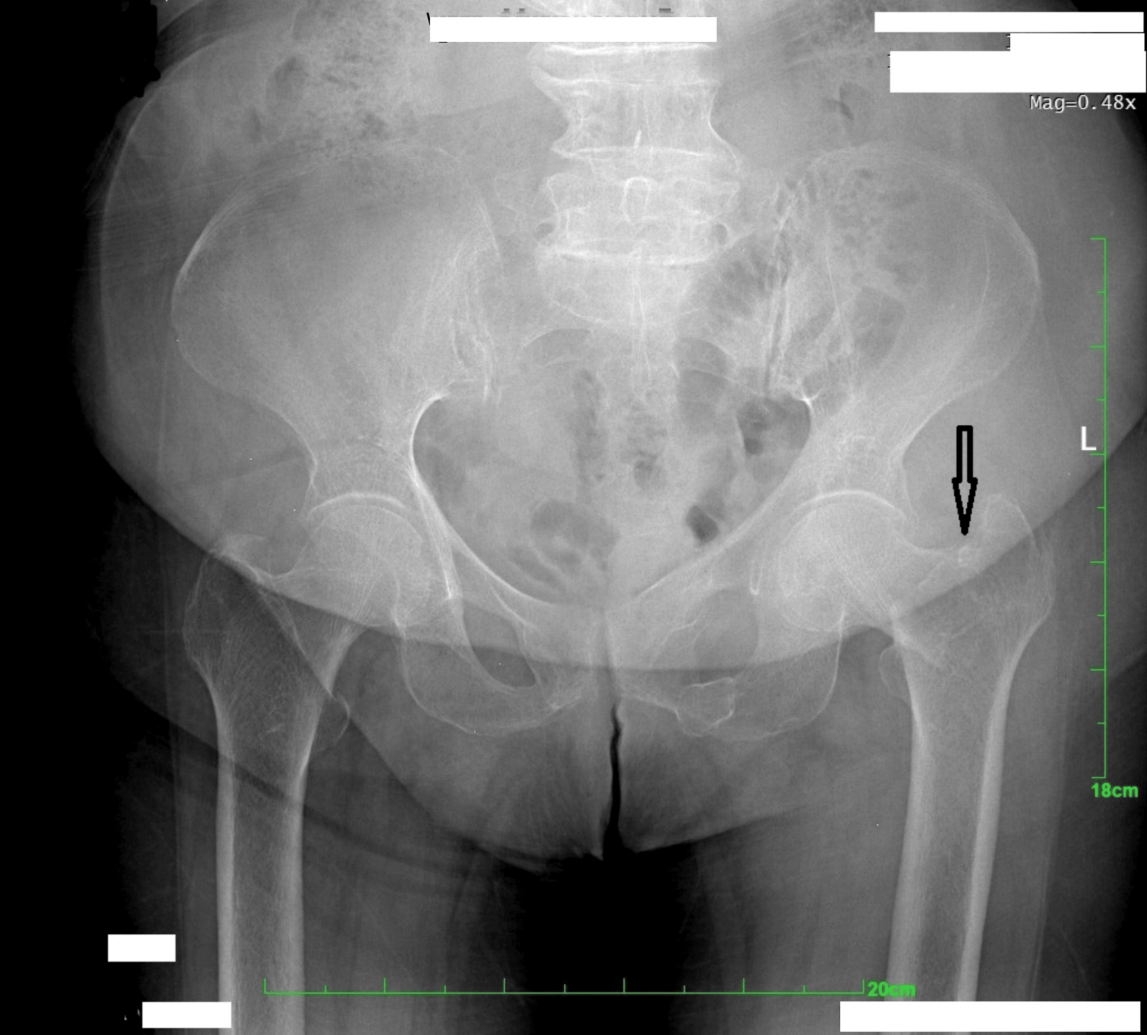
Pelvic radiograph demonstrating a left intertrochanteric femoral fracture
